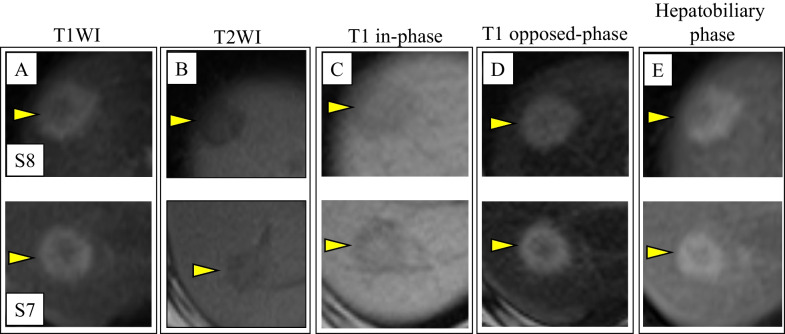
Various findings of magnetic resonance imaging (MRI) of the lesions: S8 (upper) and S7 (below) lesions by T1- and T2-weighted images (T1WI: A and T2WI: B), T1-weighted in- (C) and opposed-phase (D), and ethoxybenzyl-contrast images in hepatobiliary phase (E) are shown. The yellow arrowheads indicate the each lesion
